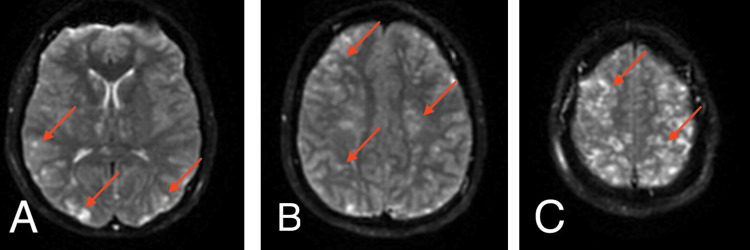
Axial DWI MRI (A, B, C), red arrows demonstrating "starry sky" infarcts, most consistent with CFE. DWI: Diffusion-weighted imaging; CFE: cerebral fat embolism
